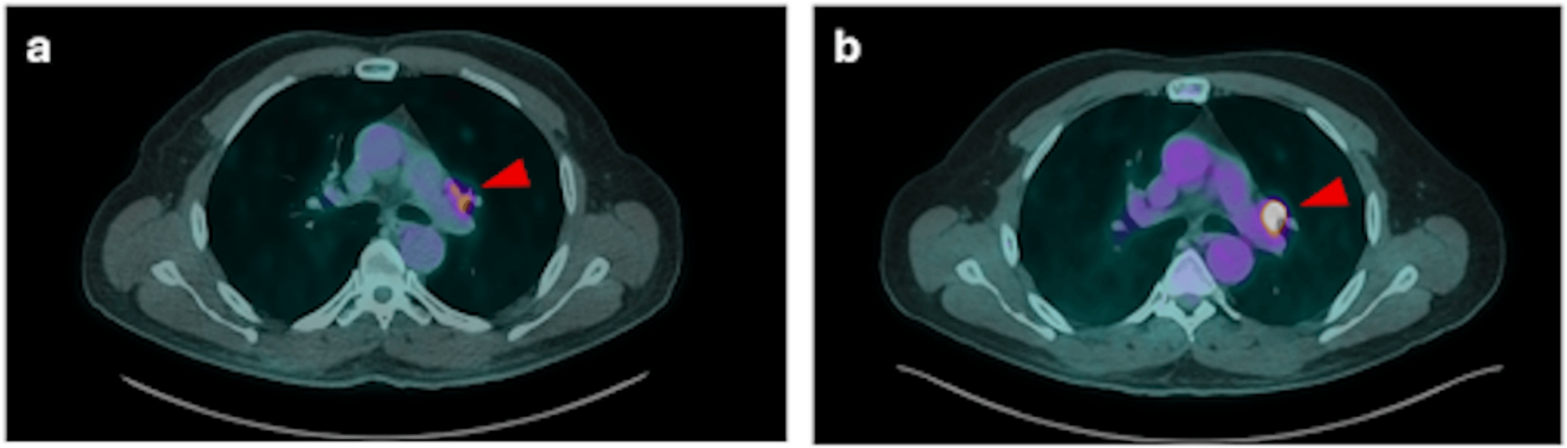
Axial FDG-PET/CT images from June 2023 (a) and July 2024 (b). An interval increase in the metabolic activity of a left hilar pulmonary nodal lesion (red arrowhead) indicates progressive disease. FDG: Fluorodeoxyglucose.
